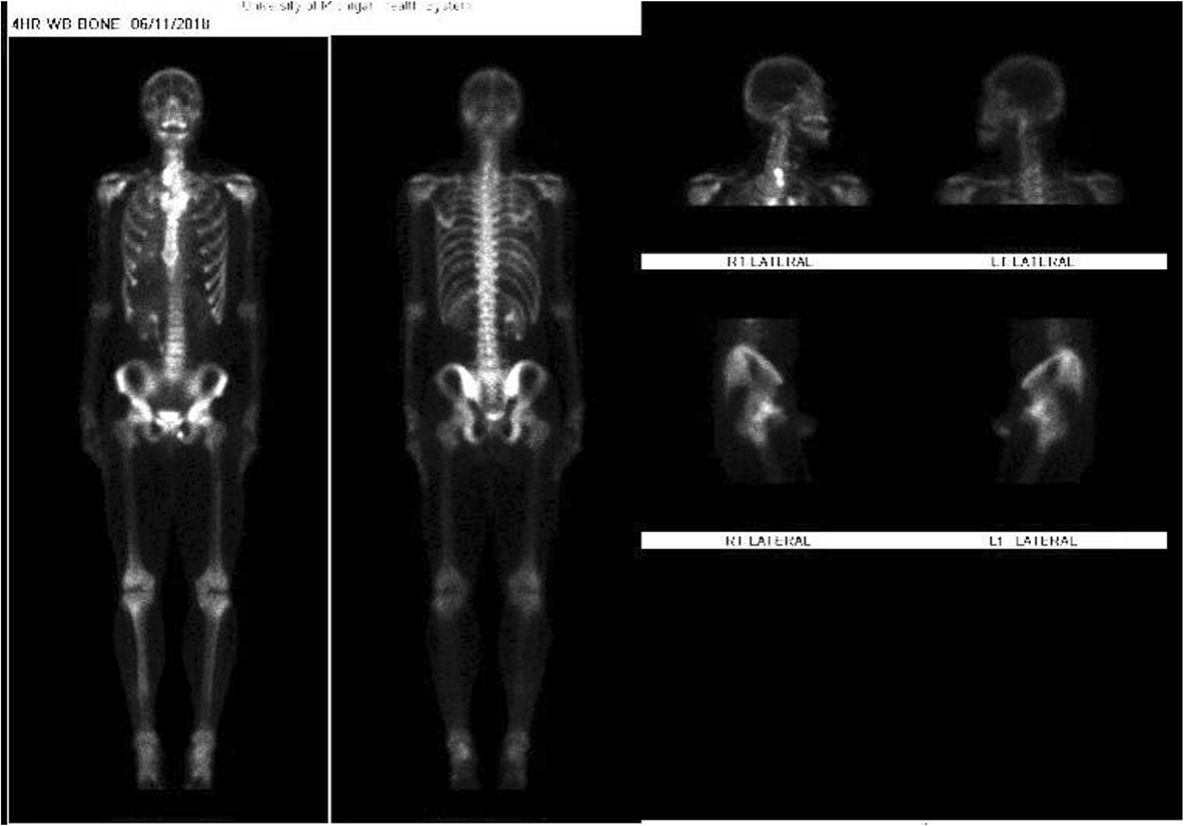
Bone Scan with SPECT/T showing lesions involving the thyroid, lung, right pleural, and right scapula
